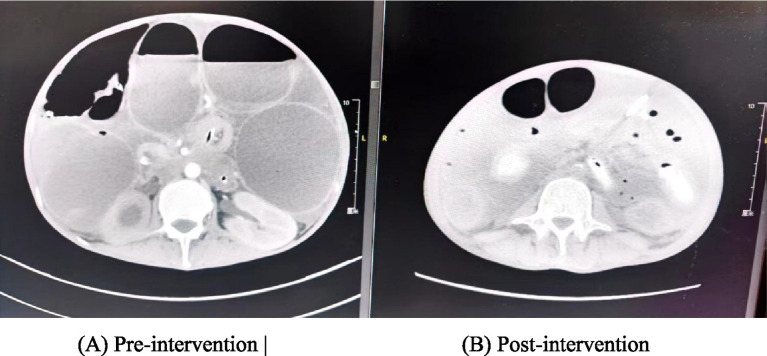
Comparative abdominal CT scans before and after treatment. (A) Pre-intervention. (B) Post-intervention.
